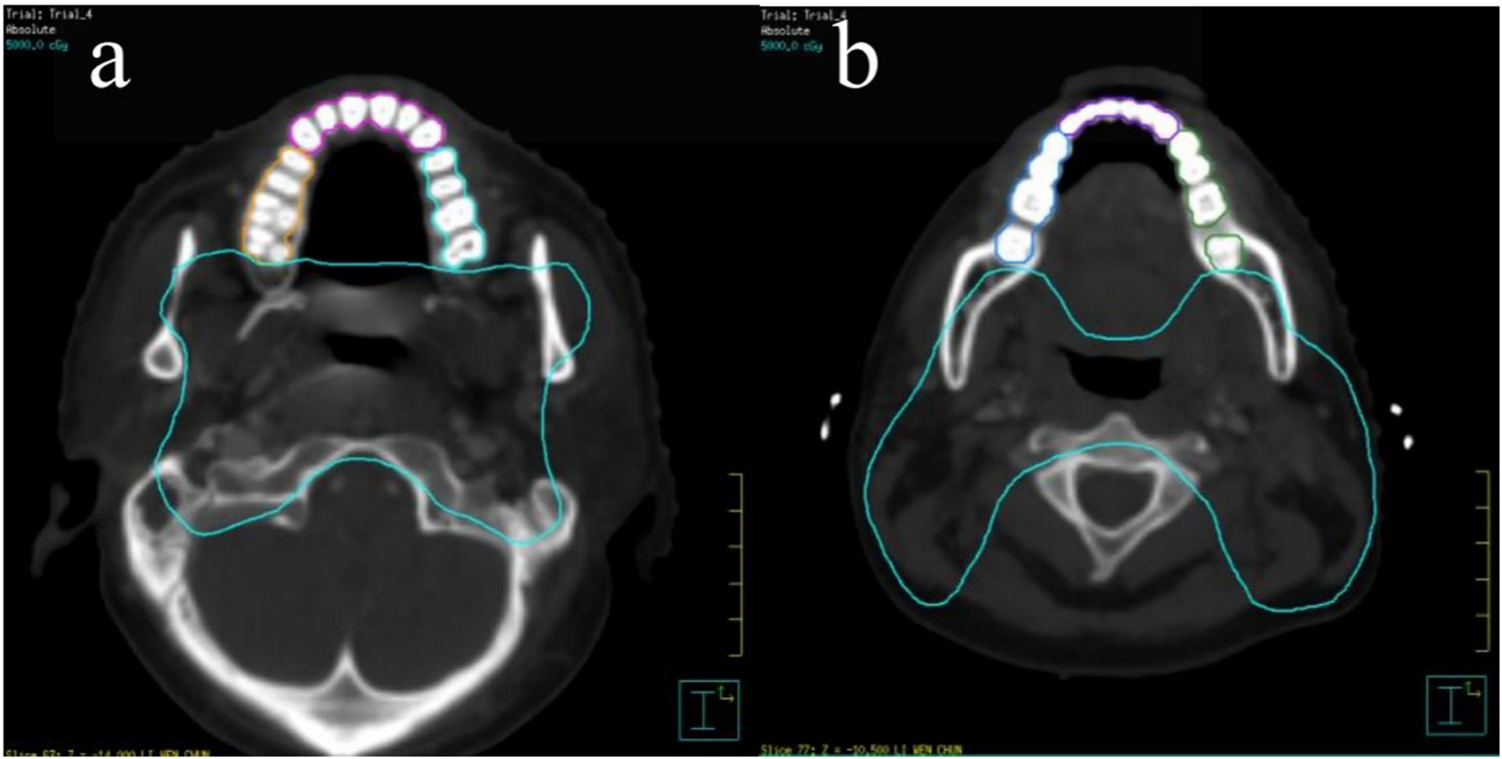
(a) Delineation of the maxillary teeth on CT images and (b) delineation of the mandibular teeth on CT images (The dose line is 50 Gy. CT: window: 1,500, level: 800.).
